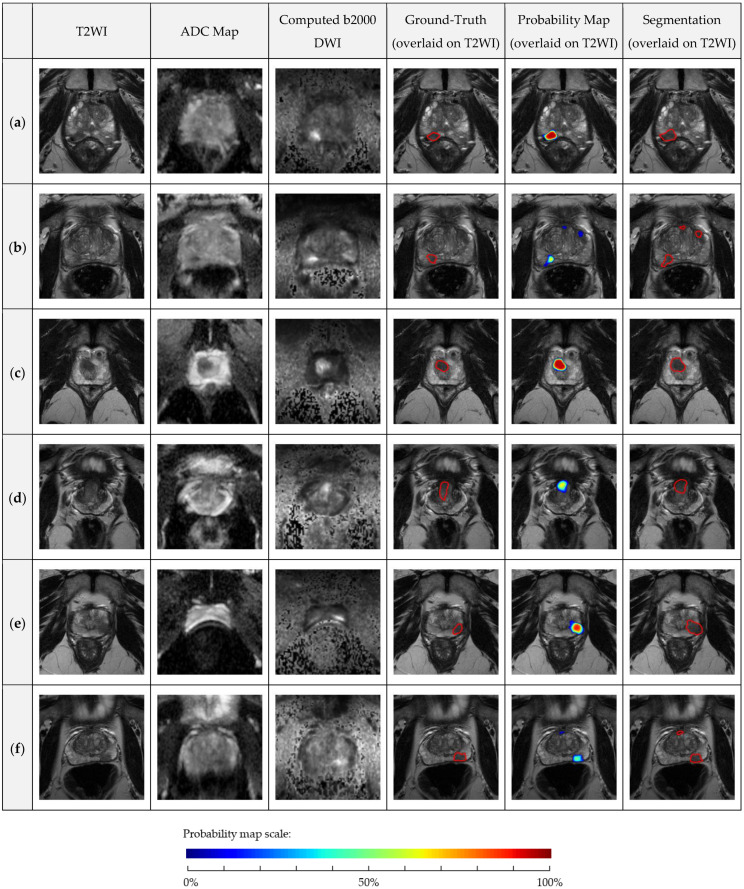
PICTURE dataset axial T2WI, ADC map, Cb2000 DWI, ground-truth lesion contour overlaid on T2WI, probability map overlaid on T2WI, and segmentation overlaid on T2WI: (a) 79-year-old man, PSA 12.57 ng/mL, midgland PZ GS 4 + 3 lesion, Likert 5, AutoProstate probability of CSPCa 100%; (b) 66-year-old man, PSA 7.50 ng/mL, midgland PZ GS 3 + 4 lesion, Likert 3, AutoProstate probability of CSPCa 65%; (c) 64-year-old man, PSA 10.53 ng/mL, apex CG GS 3 + 4 lesion, Likert 5, AutoProstate probability of CSPCa 95%; (d) 56-year-old man, PSA 7.91 ng/mL, base CG GS 3 + 4 lesion, Likert 4, AutoProstate probability of CSPCa 66%; (e) 60-year-old man with stable rectal gas-induced magnetic susceptibility artefact on DWI, PSA 6.15 ng/mL, midgland PZ GS 3 + 4 lesion, Likert 5, AutoProstate probability of CSPCa 88%; and (f) 73-year-old man with bowel peristalsis-induced magnetic susceptibility artifact, PSA 4.09 ng/mL, midgland PZ GS 3 + 4 lesion, Likert 5, AutoProstate probability of CSPCa 49%.
